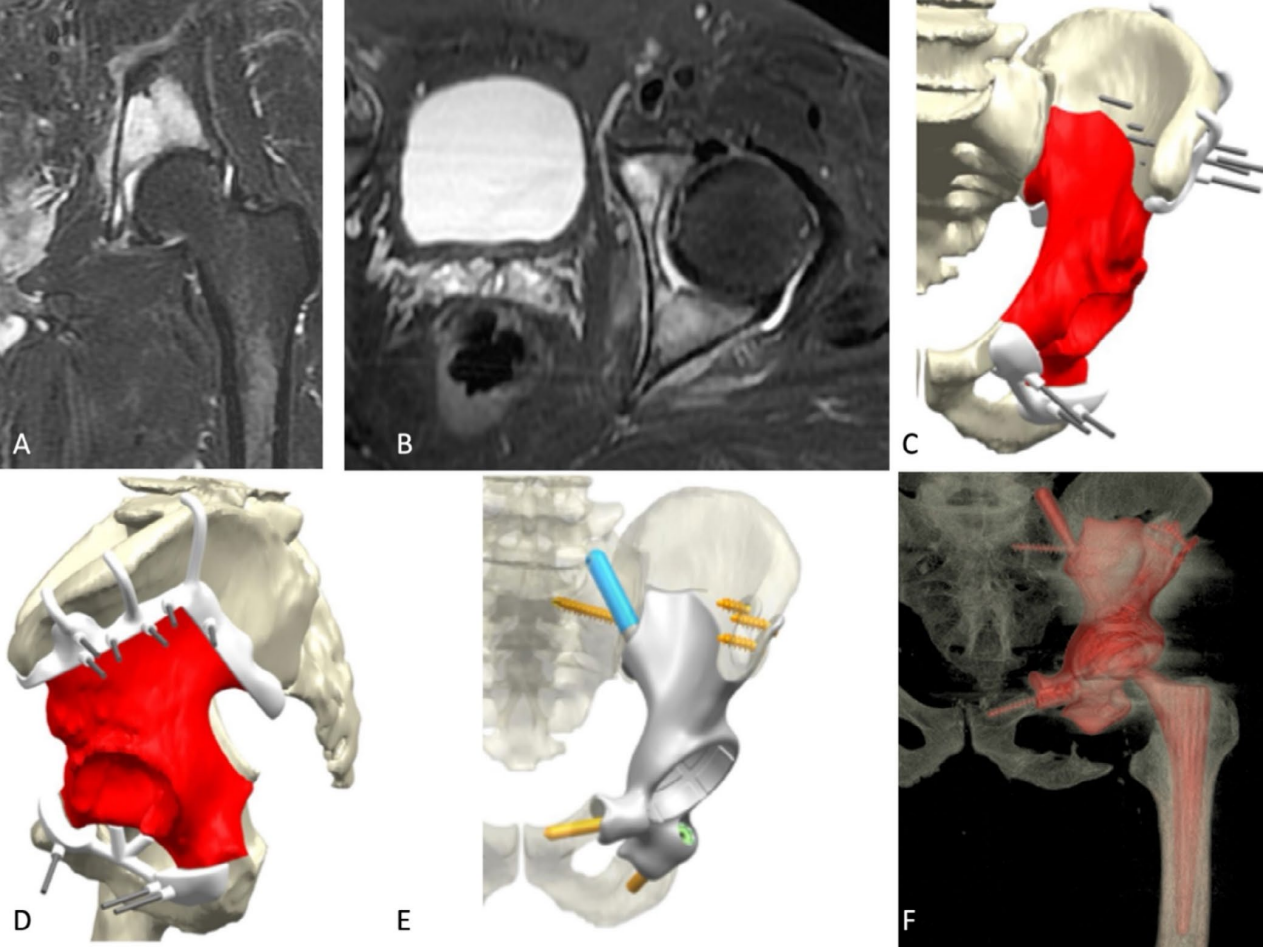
A 60‐year‐old male patient with Grade 2 chondrosarcoma. High‐intensity signals were observed in the coronal (A) and transverse (B) MRI images (T1‐fat suppression with contrast) in the left hemi‐pelvis, involving the periacetabular area but without infiltration into the hip joint. 3D computer renderings for the frontal (C) and lateral (D) views of the left hemi‐pelvis show the planned P1–P2–P3 resection highlighted [in red] and the planned 3D‐printed custom‐made cutting guide positioning and fixation [in white]. (E) 3D computer renderings post‐tumor resection depict the insertion of the 3D‐printed prosthesis and its fixation points. The actual cutting points were confirmed using a 3D‐printed bone model. The fixation points for the cancellous screws and stems were also determined, allowing for the calculation of the appropriate lengths for drilling and the sizes of the planned screws or stems. (E) Postoperative CT 3D reconstruction after the tumor resection and prosthetic reconstruction. It should be noted that the screw on the ischial branch is not included in the figure, as resection was extended intraoperatively and fixation omitted in that region, following soft tissue disease progression observed during chemotherapy.
